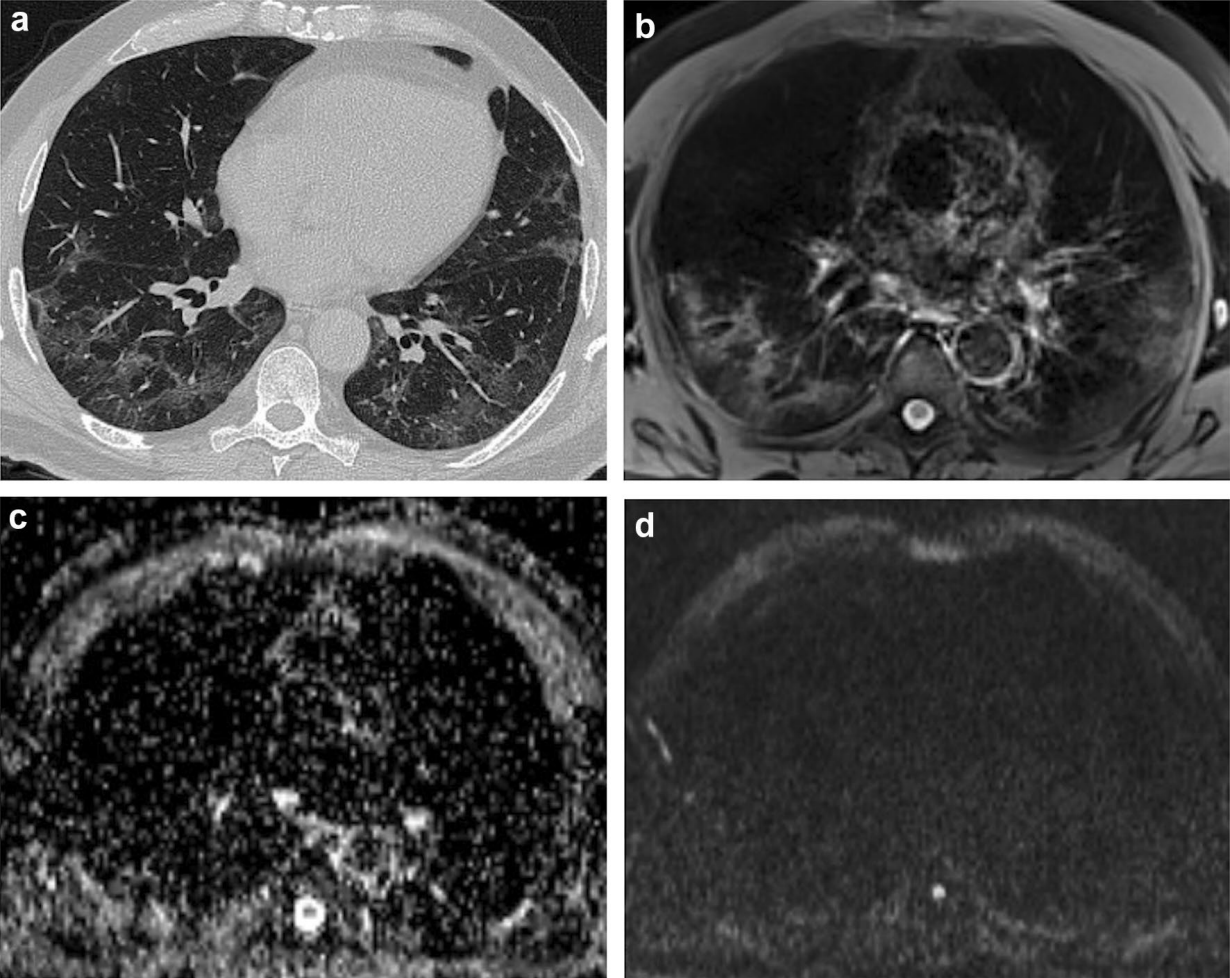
Interlobular septal thickening on ground-glass opacity. 56-year-old male. a End-inspiratory axial CT after 1 month from discharge, b Free-breathing PD-weighted axial image after one month from discharge, c Apparent Diffusion Coefficient Map, and ﻿d diffusion-weighted imaging (DWI) free breathing (*b* = 1000 s/mm^2^). Note areas of interlobular septal thickening superimposed to a GGO, on both the right and the left lower lobes that do not show restricted diffusion on DWI nor hyperintensity on ADC map
